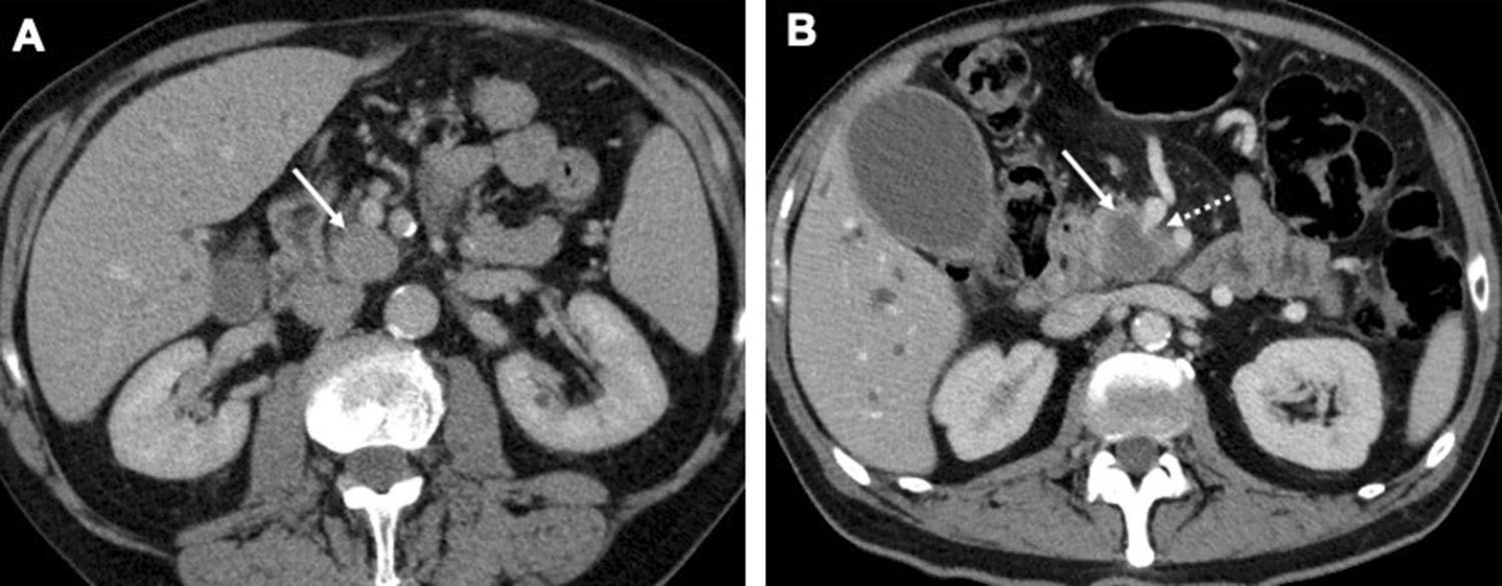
Comparison of CT features of ITPN with associated invasive carcinoma and PDAC. (A) Axial CT scan in the portal venous phase showing ITPN lesion in the pancreatic head (arrow) with well-circumscribed margins and isodense HU values. Note the preserved morphology and boundaries of the superior mesenteric vessels. (B) Axial CT scan of a different patient in the portal venous phase showing PDAC in the same location (arrow) showing unsharp margins, hypodensity und classical tear-drop sign of superior mesenteric vein (dotted arrow), suggesting its infiltration.
